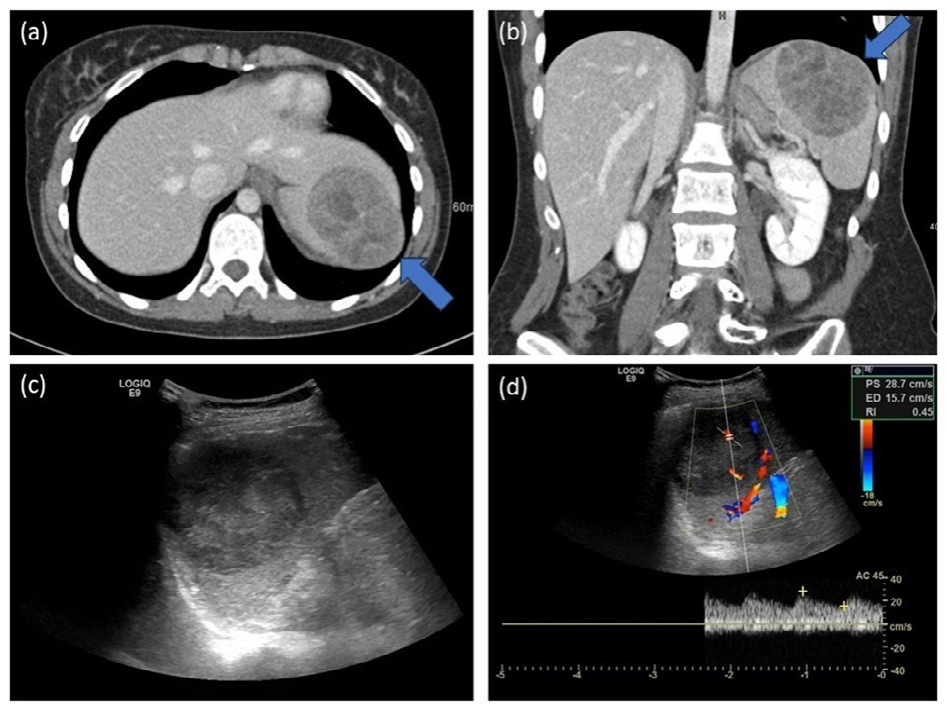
Splenic metastasis. (a, b) Abdominal CT. A sharply defined mass (5.5 × 7.2 × 5.8 cm), globally hypodense and inhomogeneously contrast-enhancing, located cranially and subcapsular in the spleen. Notice the bulging of the diaphragm (arrows), without signs of pleural/pulmonary invasion. (c, d) Abdominal US. A sharply defined, heterogeneous mass (5.5 × 7.2 cm) located cranially and subcapsular in the spleen. Colour Doppler shows present yet not pronounced vascularization.
